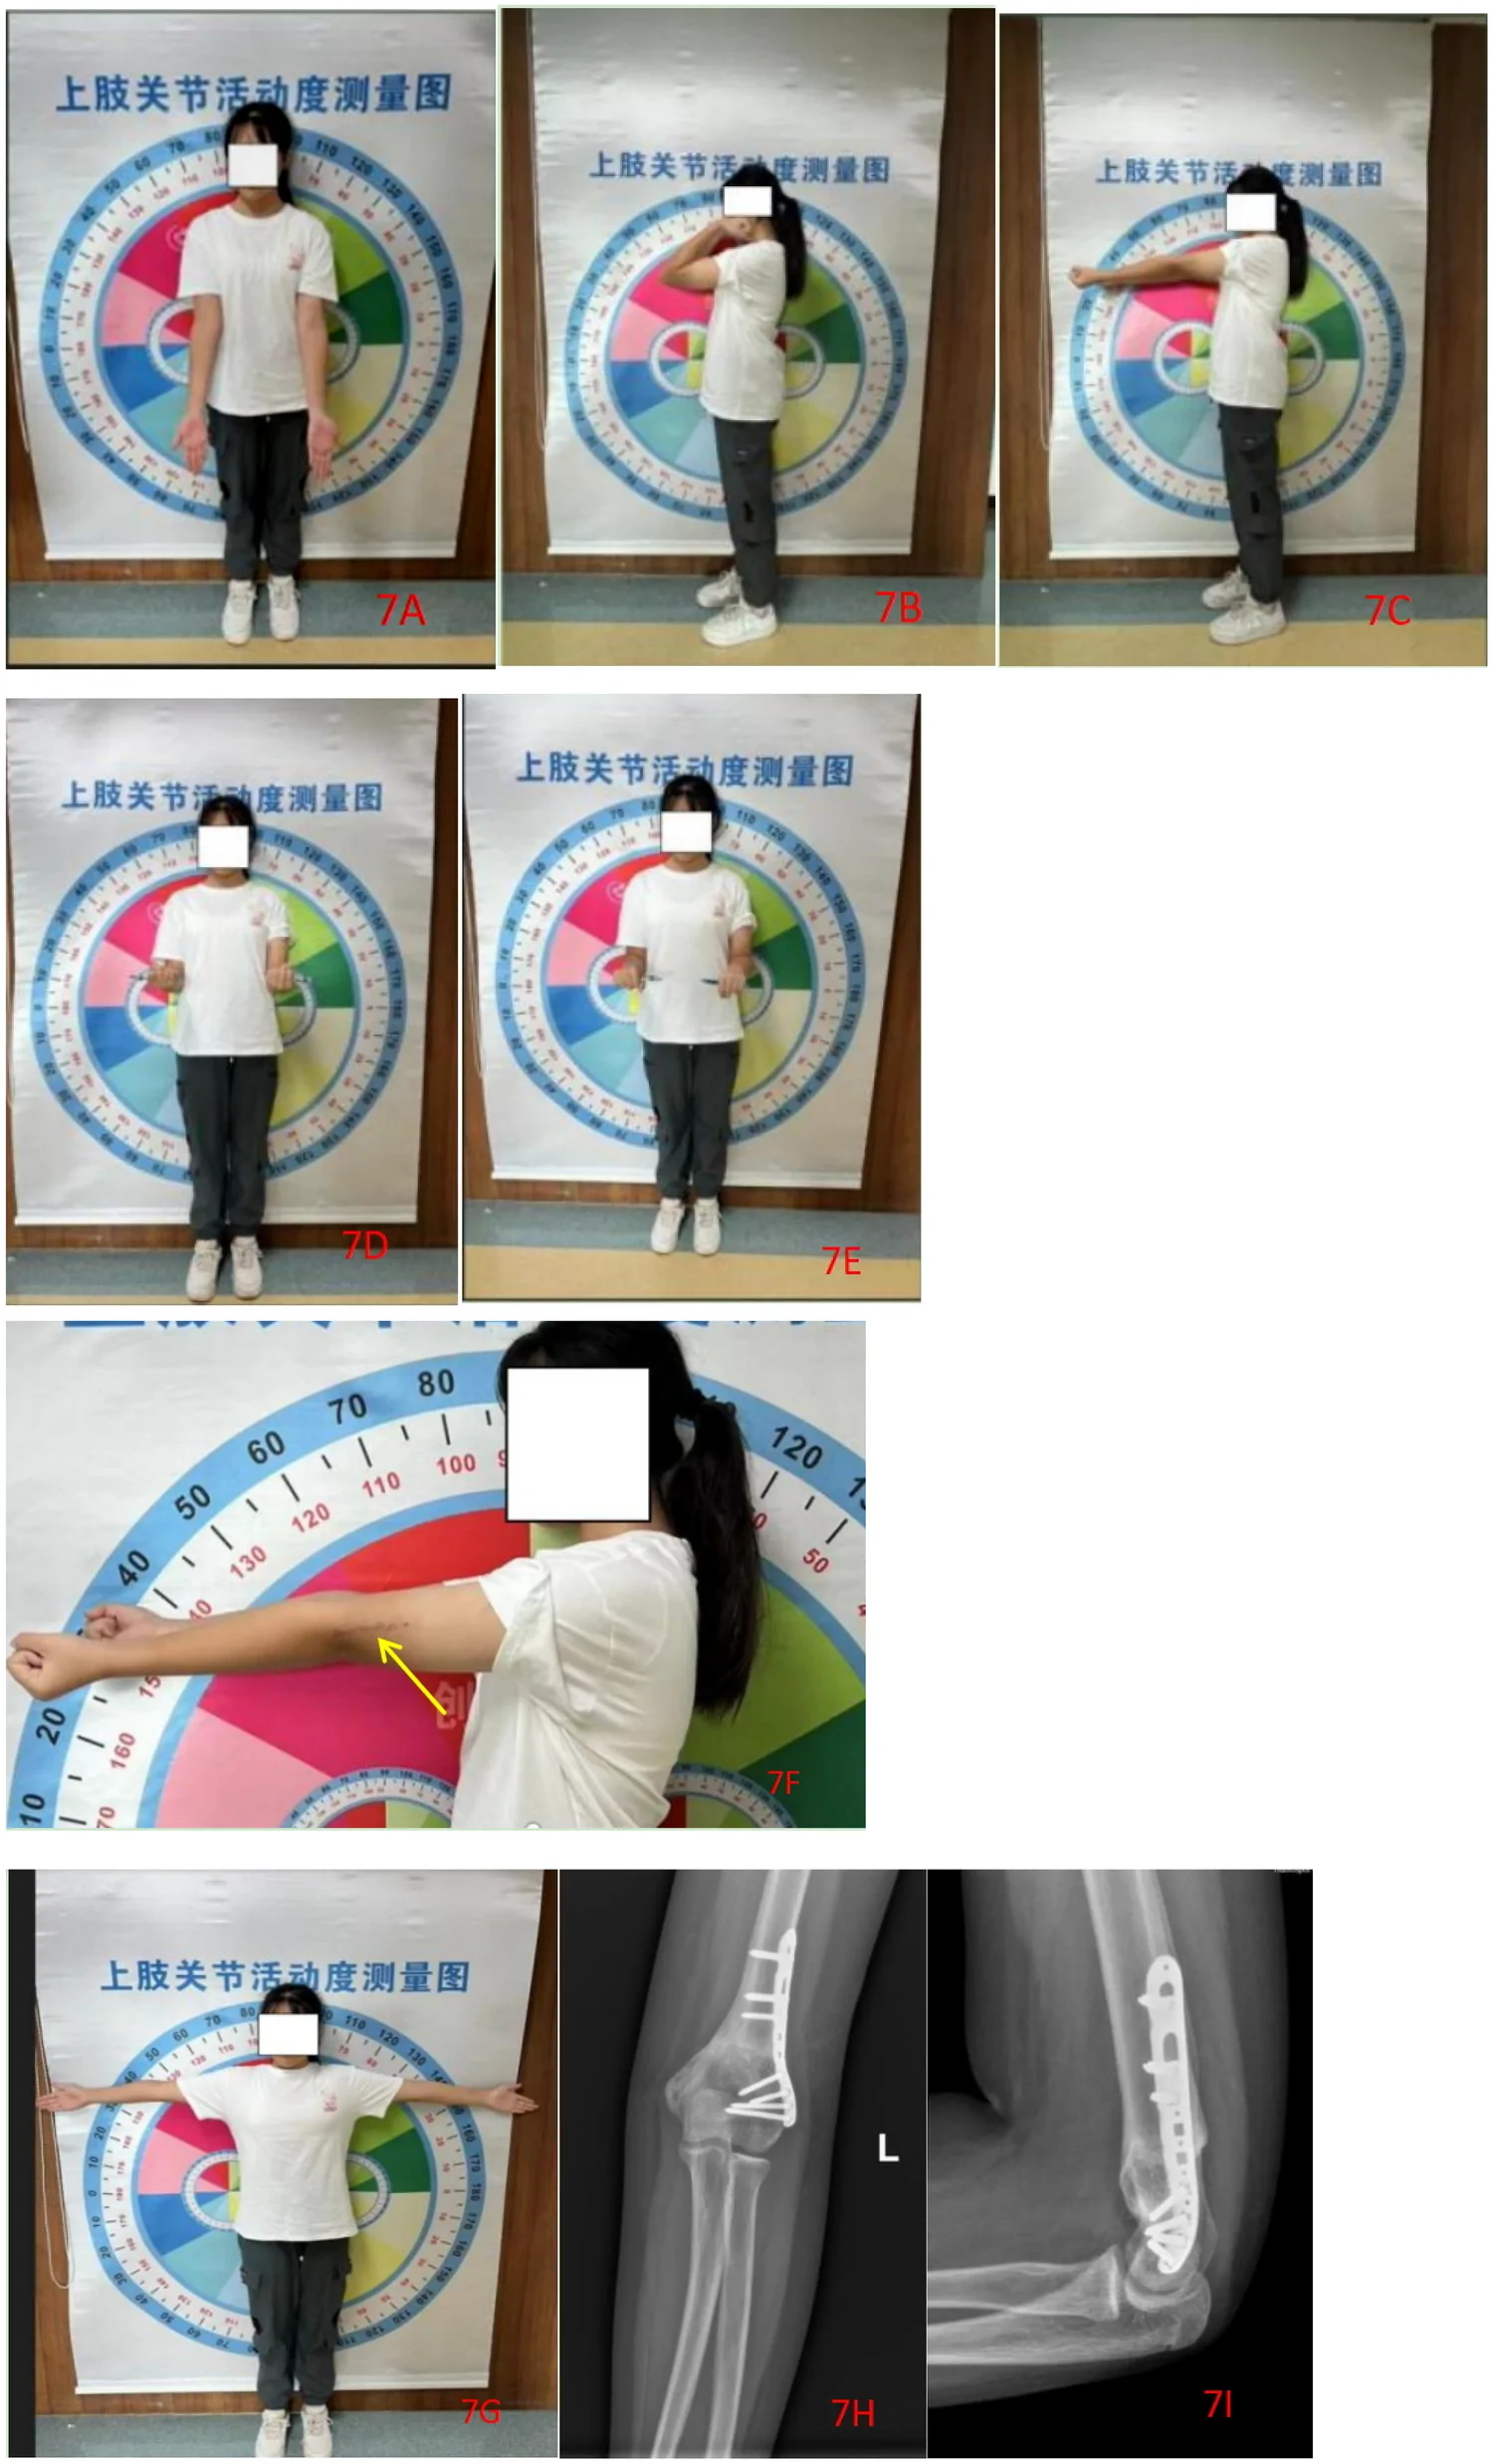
One-year postoperative follow-up. (A–E) Clinical examination showing nearly identical bilateral elbow alignment and full range of motion. (F) Well-healed 8 cm lateral incision. (G–I) Postoperative photographs and radiographs confirming accurate correction comparable to the contralateral normal sid.
